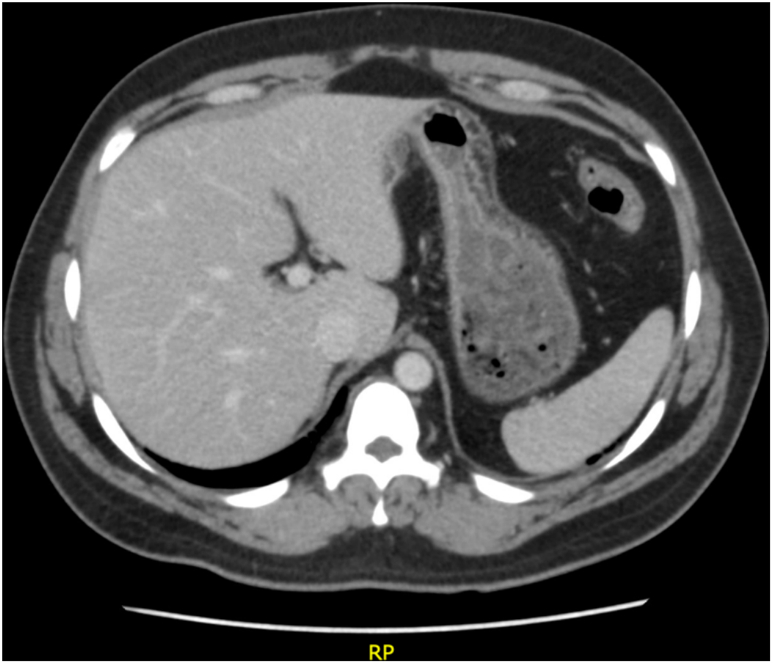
Abdominal CT scan showing complete resolution of the liver cyst.
